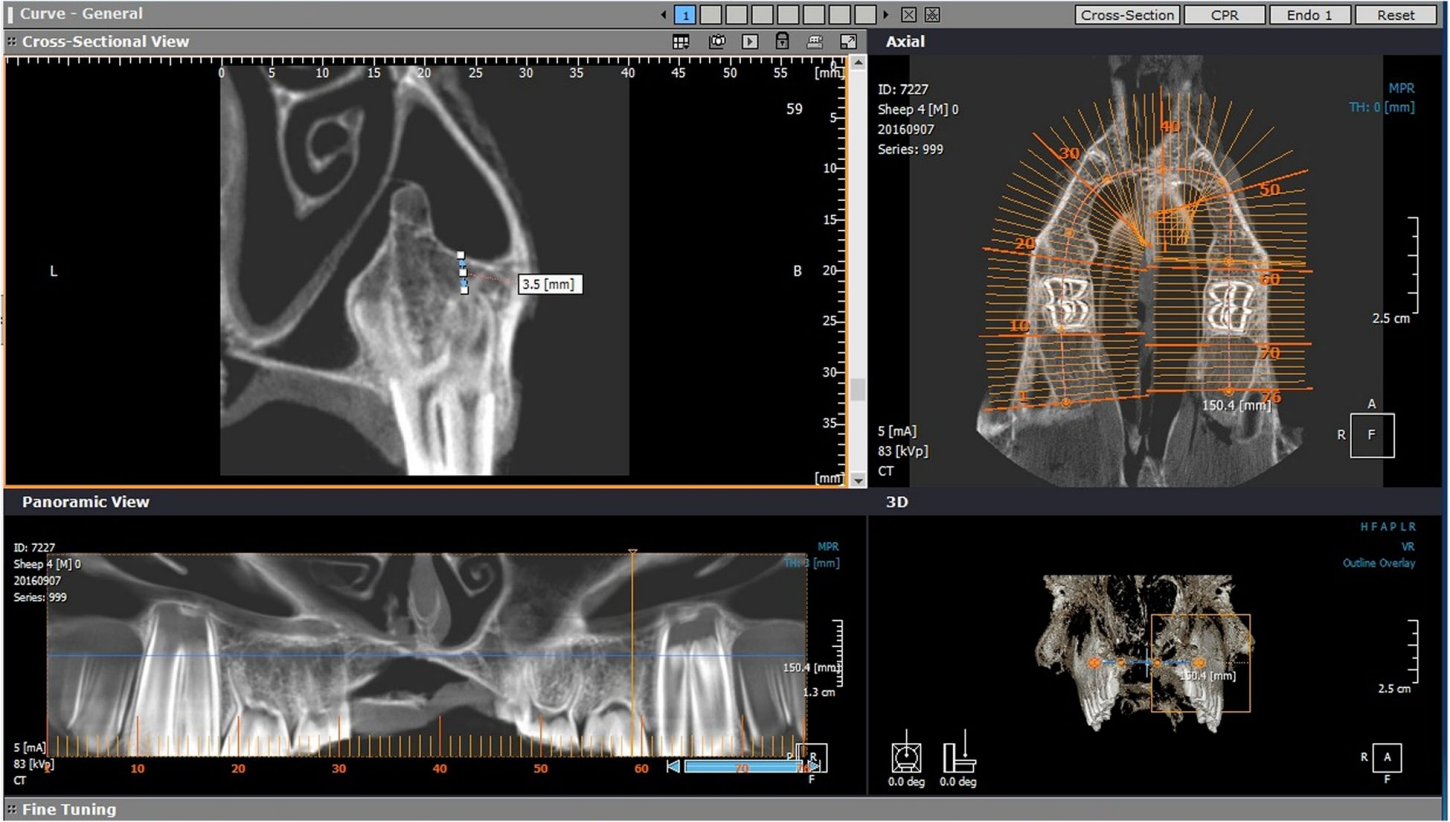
Determination of the remaining bone height (RBH) on the CBCT image
